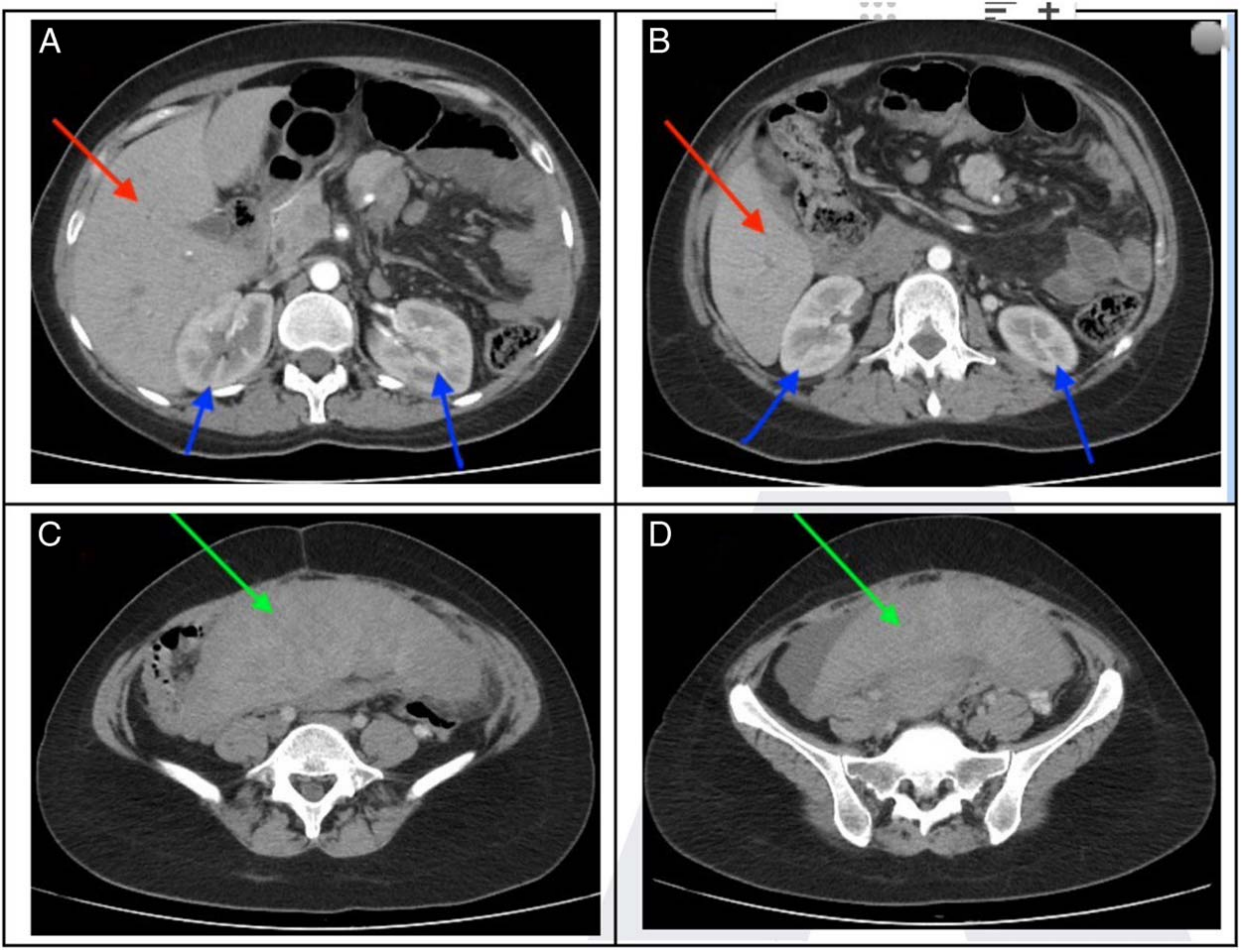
The axial CT image from A to D reveals that the spleen (green arrow) is not located in its usual position. It is situated at a lower level of the kidneys (blue arrow) and in the pelvic cavity. Liver in red arrow.
